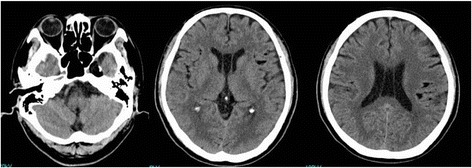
Axial unenhanced computed tomography scans showing low-density lesions in the left cavernous sinus and subarachnoid space
